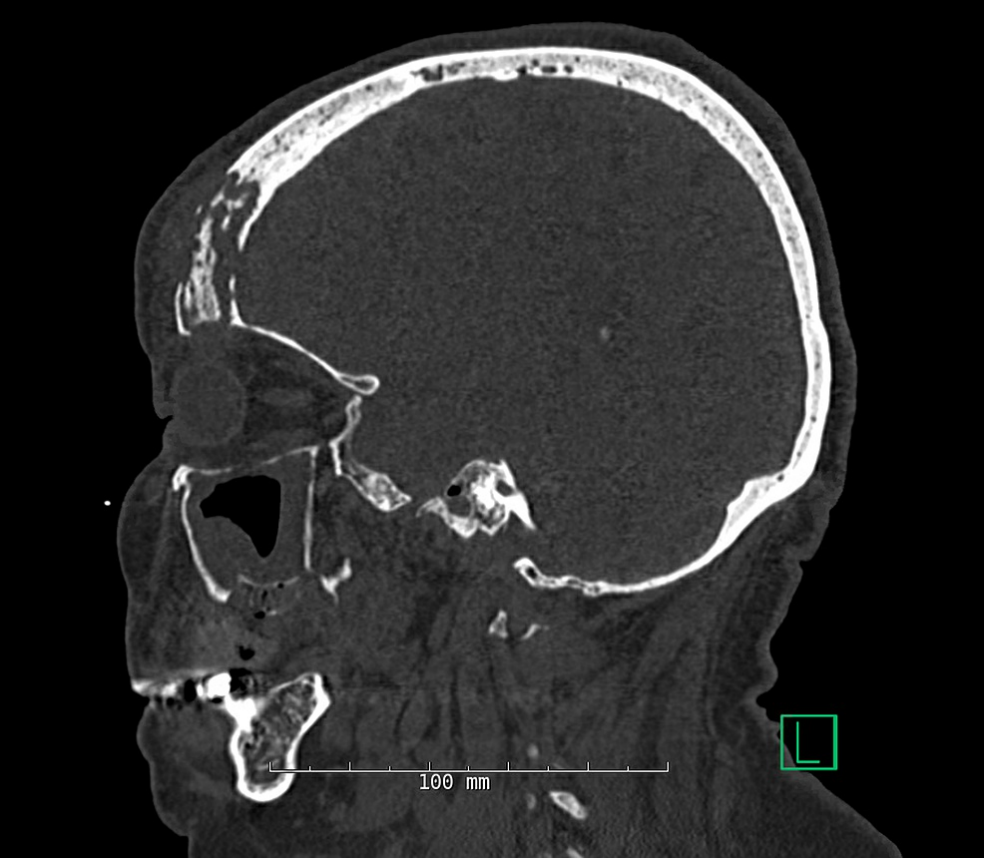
CT Head With Dehiscence of Inner Table
